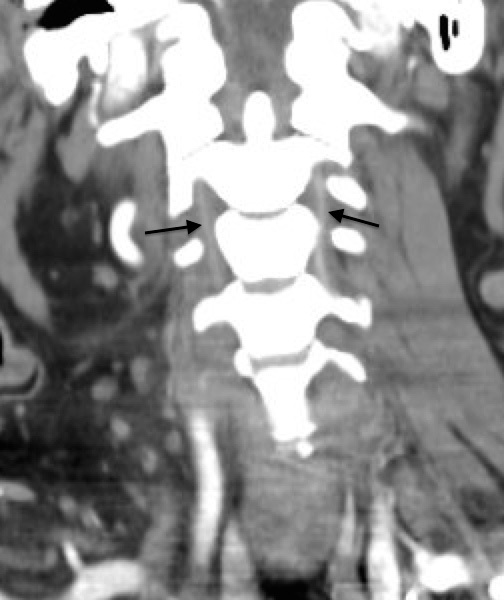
Coronal computed tomography image showing decreased vertebral artery flow bilaterally.
